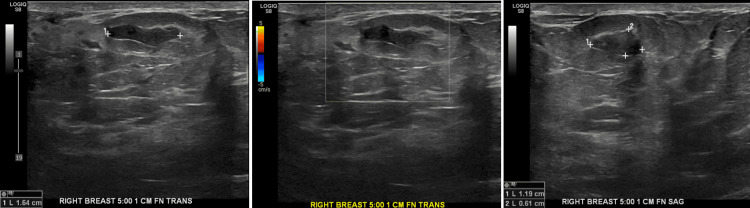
Follow-up ultrasonography at 15 months after initial mammography Ultrasonography of the breast mass in the right breast at 5 o'clock. The same oval mass was stable at 1.6 x 0.6 x 1.2 cm.
